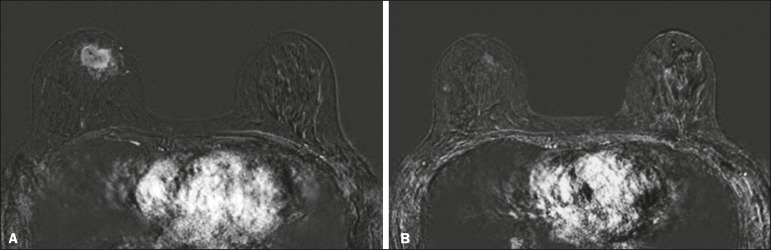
Example of a true-negative result. Pre- and post-NAC MRI (A
